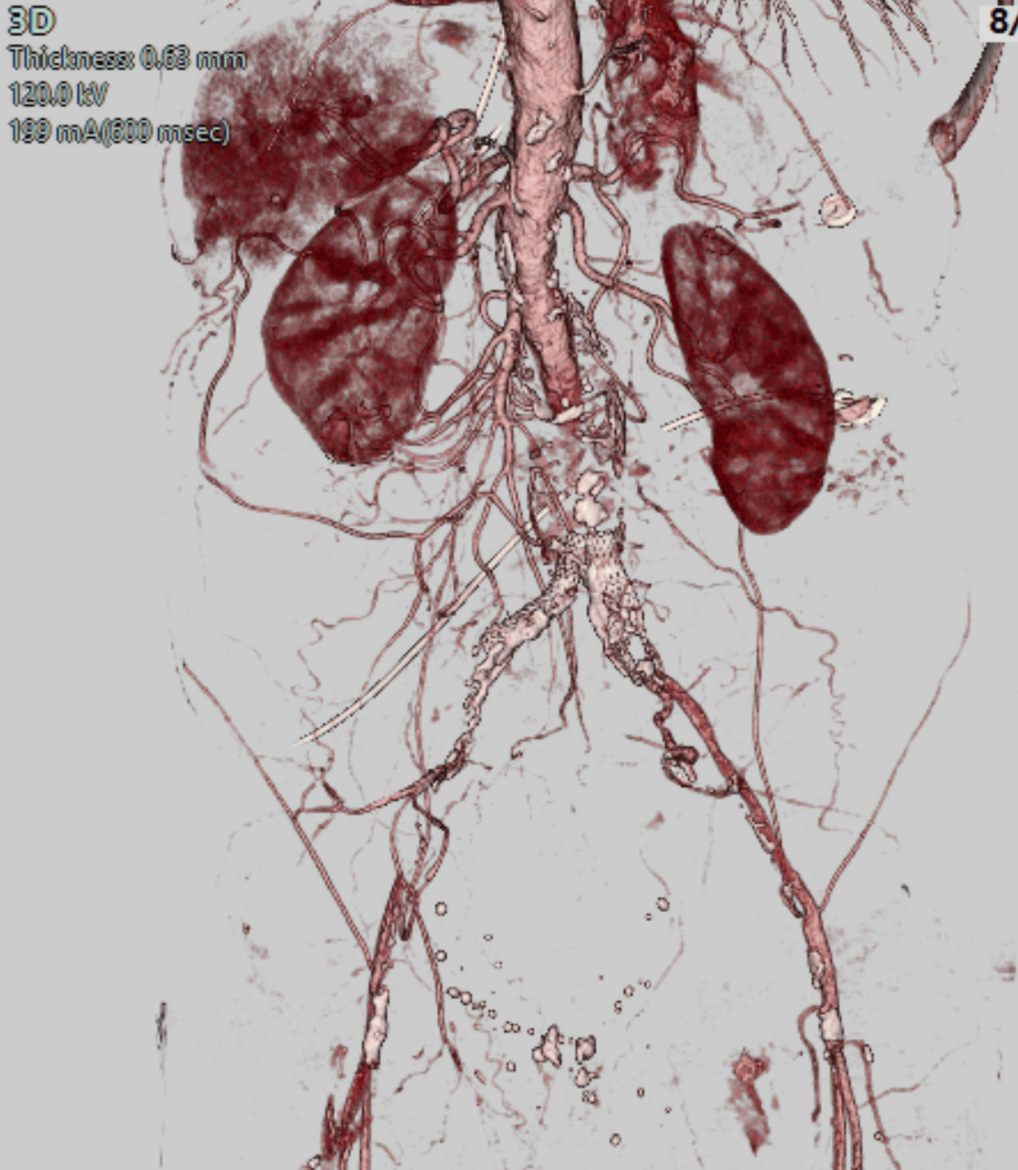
Three-dimensional reconstruction of CTA CTA: computed tomography angiogram
